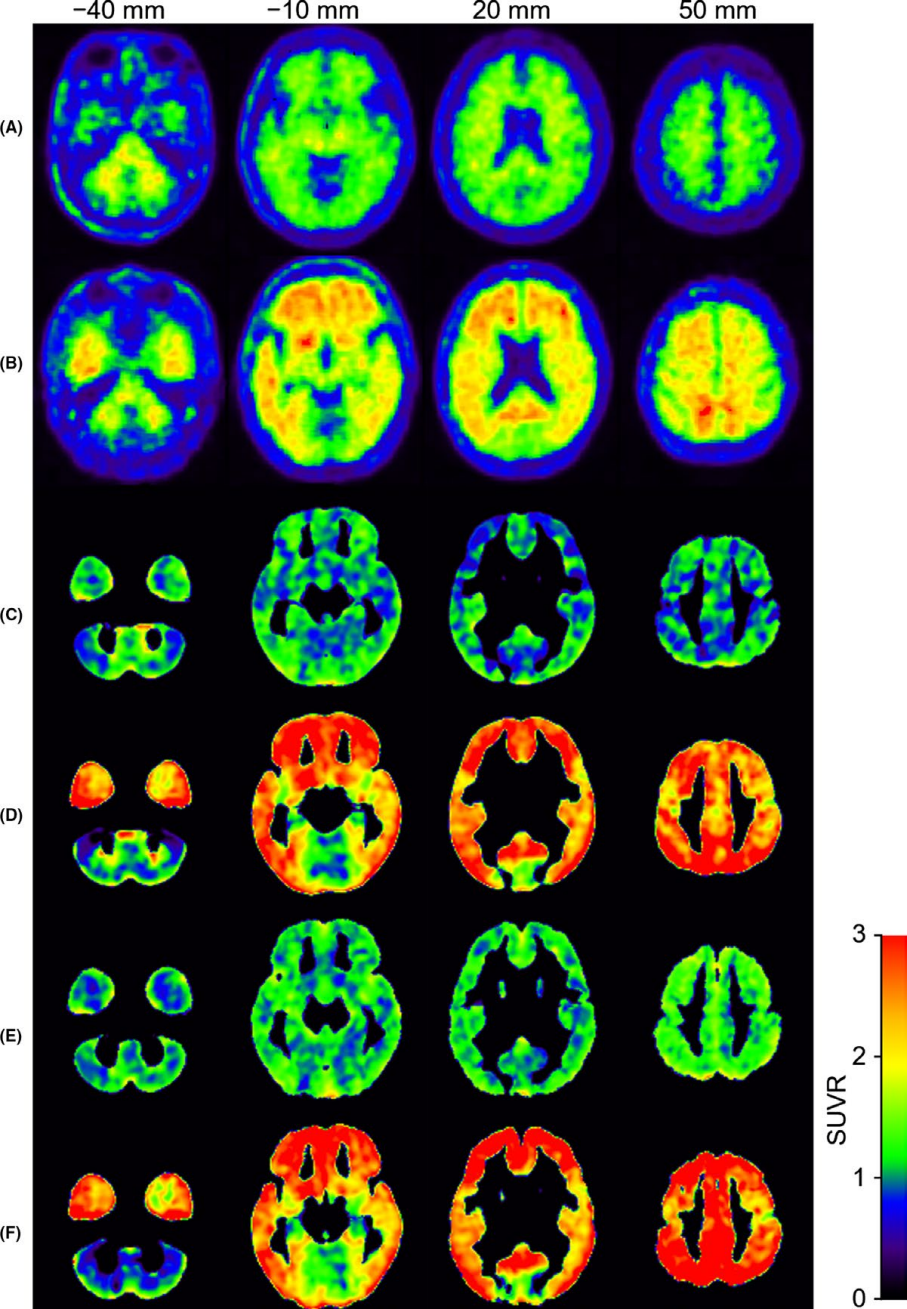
Examples of the SUVR cross‐sectional images generated from a normal volunteer (rows A, C, and E) and an Alzheimer disease (AD) patient (rows B, D, and F). Rows A and B correspond to standard uptake volume ratios (SUVRs) without partial volume correction (PVC). Rows C and D correspond to SUVRs with CT‐based PVC. Rows E and F correspond to SUVR with MRI‐based PVC. The columns, left to right, correspond to axial images at the levels of −40, −10, 20, and 50 mm, respectively. The signal intensities range from 0.0 to 3.0
